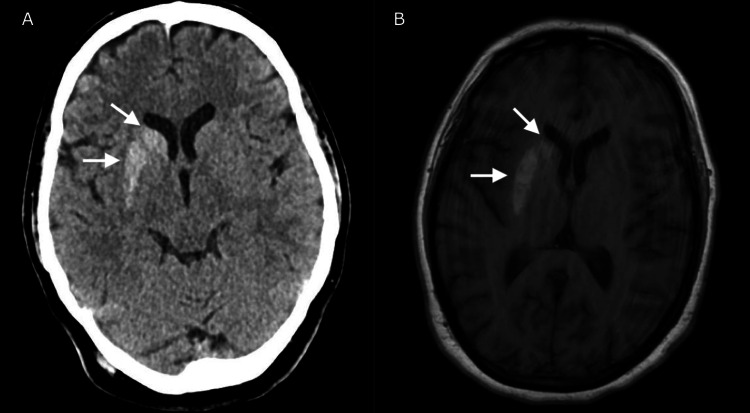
CT head (A) and MRI brain (B) showing a hyperdense and hyperintense lesion respectively in the right basal ganglia.
